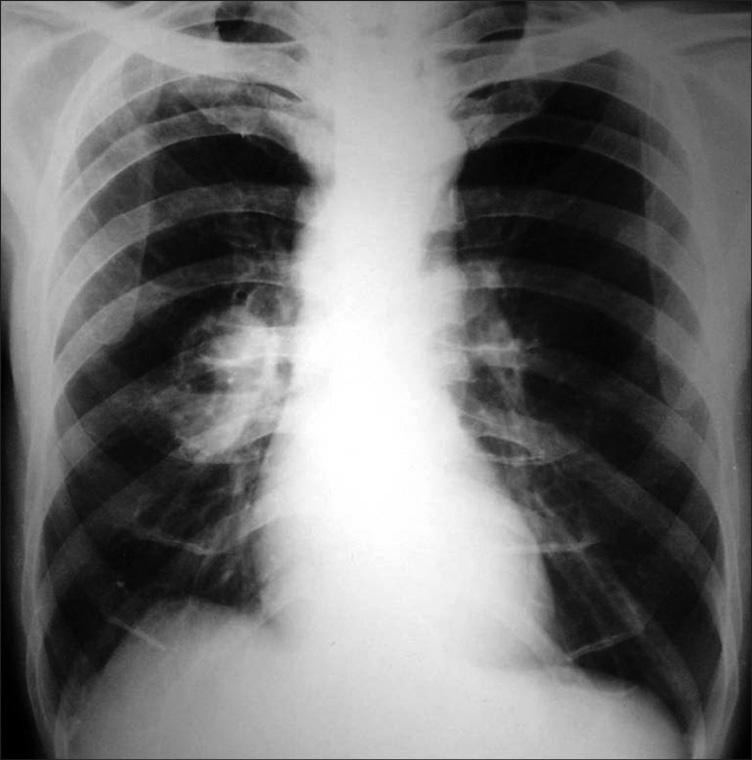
Chest x-ray showing right parahilar cavitary lesion
